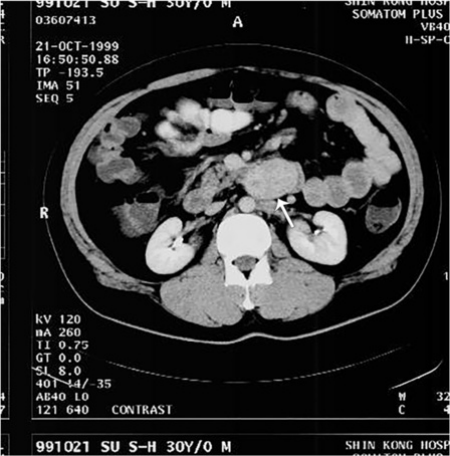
Contrast abdominal computed tomography showing a 7 cm × 6 cm × 4 cm mass in the proximal jejunum (*white arrow*)
